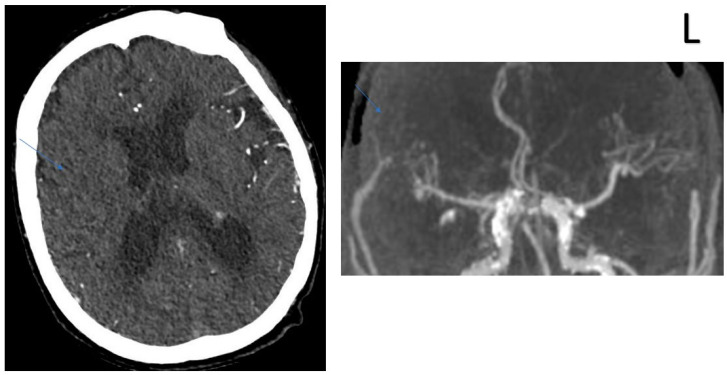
COLLATERAL SCORE: 0–80-year-old female patients. Right MCA infarction. NIHH Score: 23. CT angiography was performed 3.5 h after the acquisition of clinical findings. Left image, “Subtracted MIP image obtained from arterial phase images”. Right image, “Raw image in the axial plane obtained in the arterial phase after contrast injection”. Arrows represent collateral score area.
